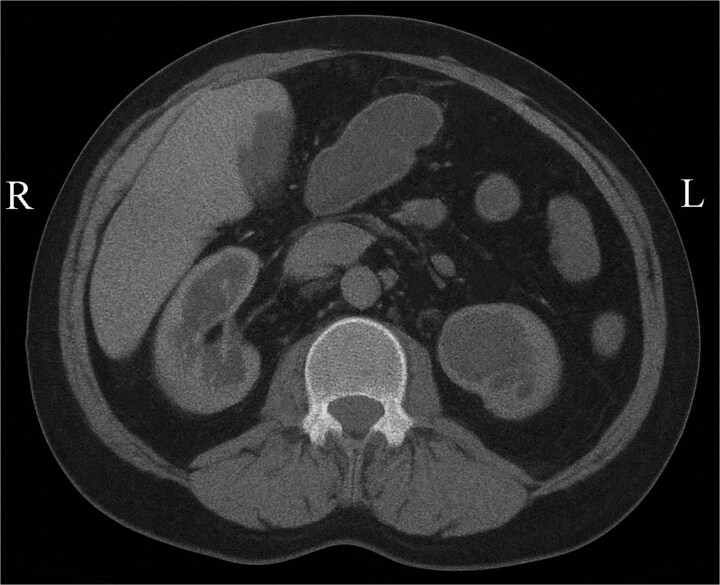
Axial computed tomography image of the abdomen demonstrating renal anomalies.
